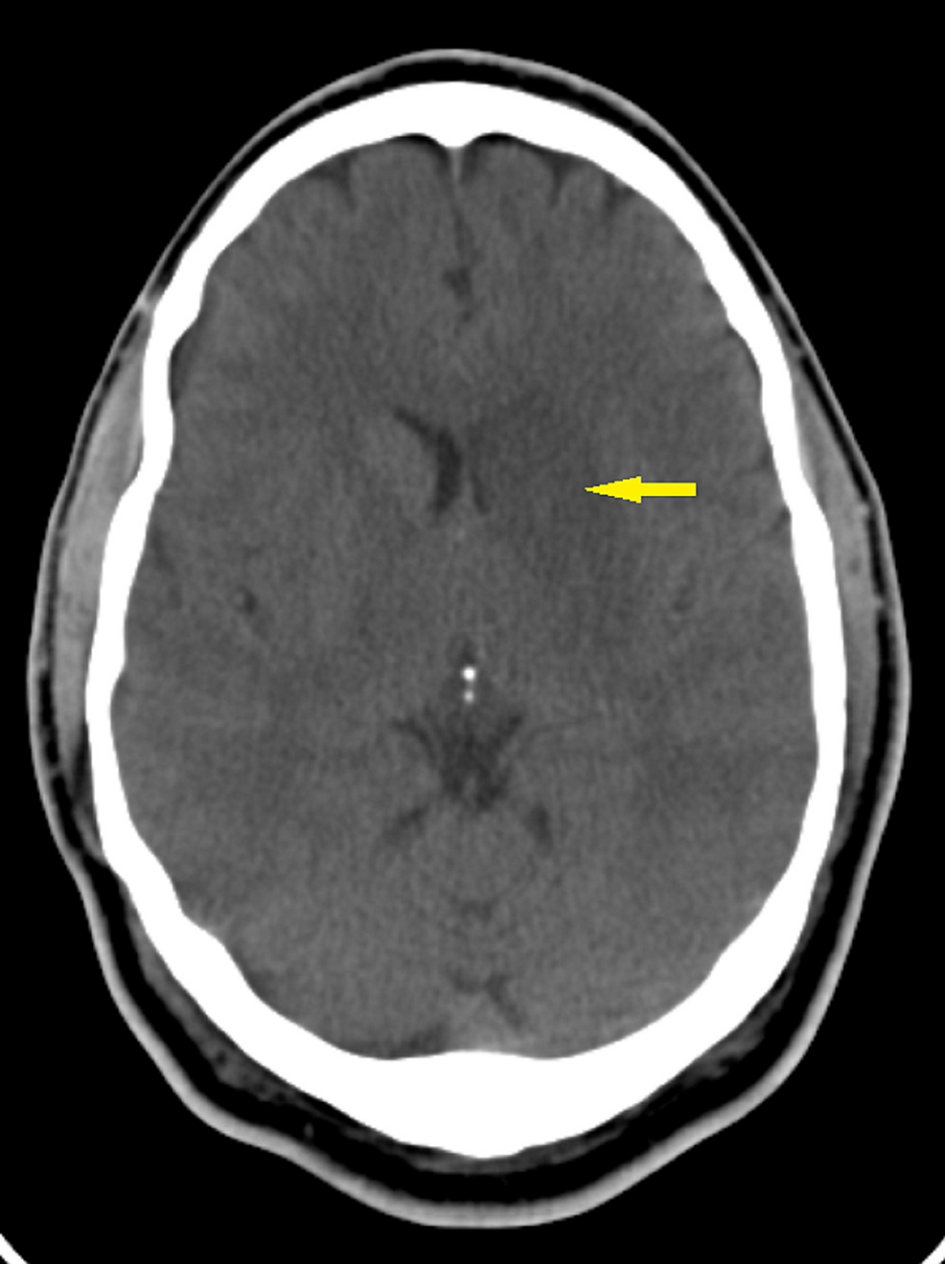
CT of the head showing acute infarct involving the left basal ganglia and head of the left caudate (12/2018)
